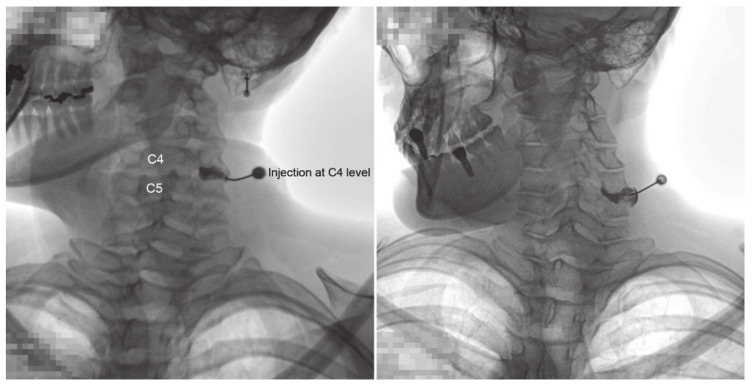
Arthrogram X-ray images showing the normal cervical facet joint flow.
